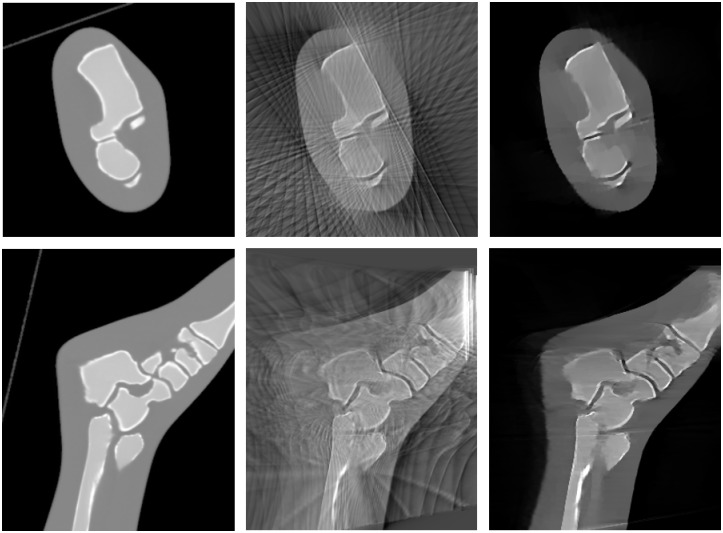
Axial and coronal views of the CT volume acquired on the helical scanner (left) and of the reconstruction of the limited data with 25 projections obtained on the C-arm with FDK (center), and with SCoLD using the acquired mask (right).
